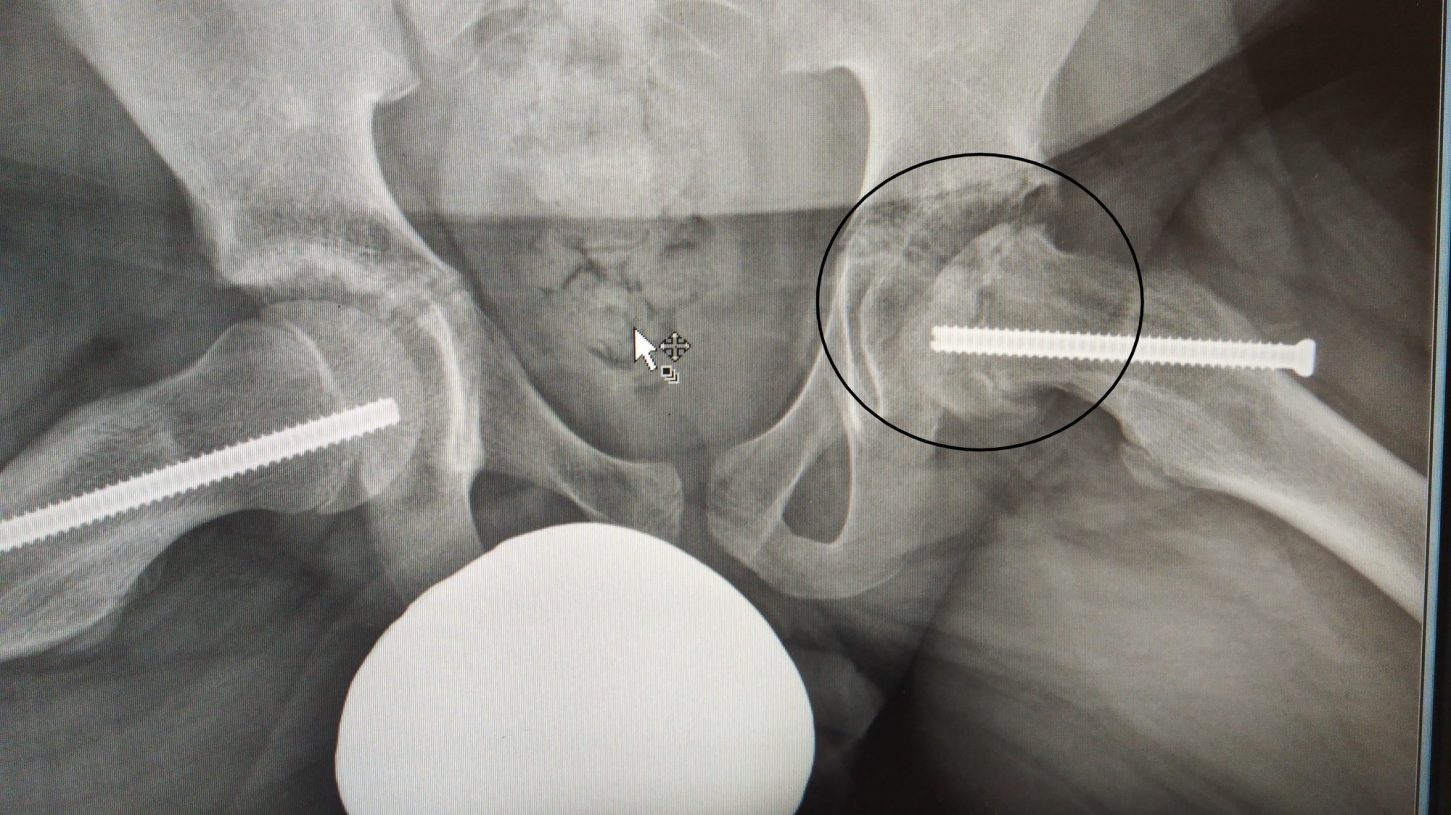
Frog lateral X-ray. Frog lateral X-ray pelvis, the encircled left hip showing avascular necrosis.
